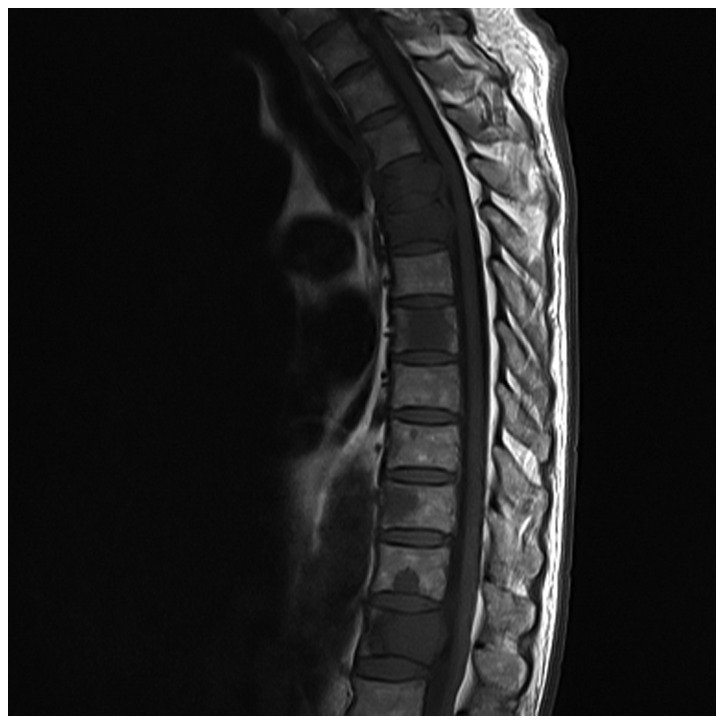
Magnetic resonance image of the vertebrae nine months following surgery revealed multiple vertebral metastases; the metastatic lesion at the 4th thoracic vertebra had compressed the spinal cord.
